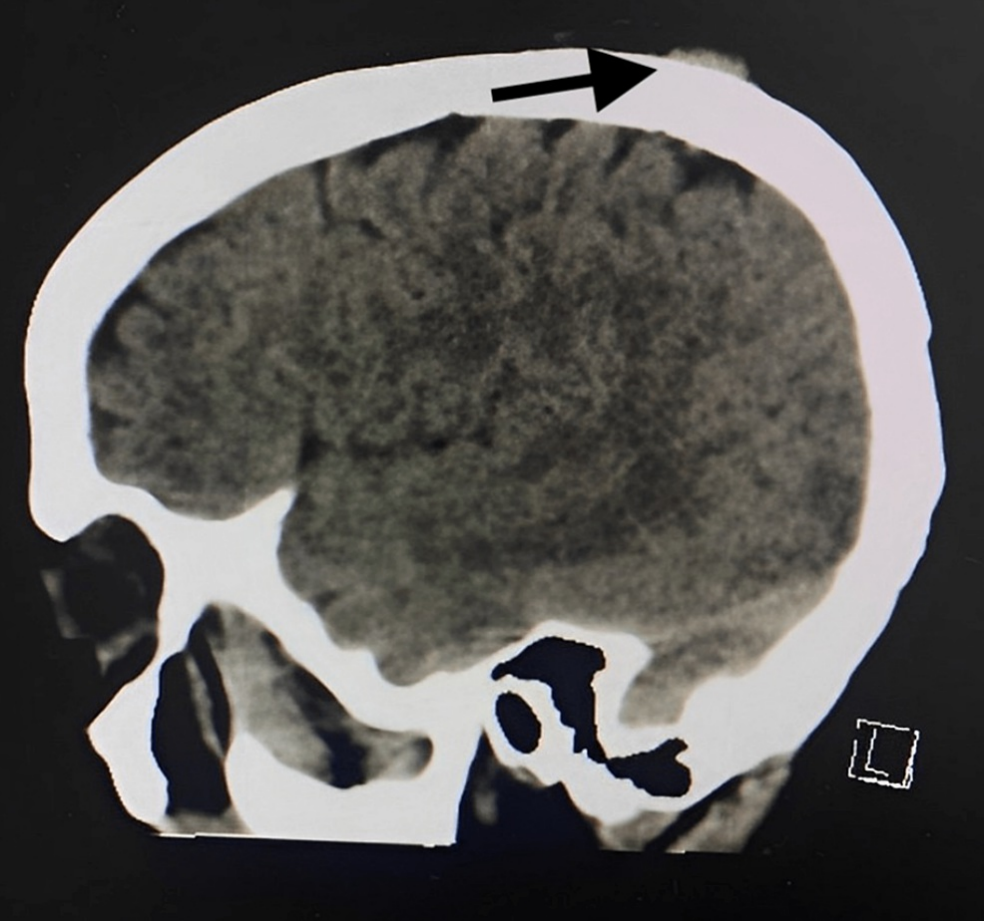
CT scan of the brain without contrast (sagittal view) showing right scalp swelling without bone destruction or intra-cranial extension
